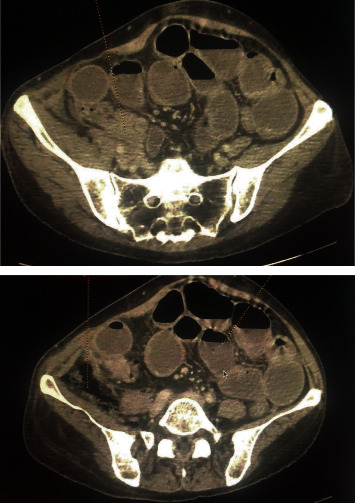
CT scan showing cecal tumor with small bowel distension and air in the retroperitoneal space.
